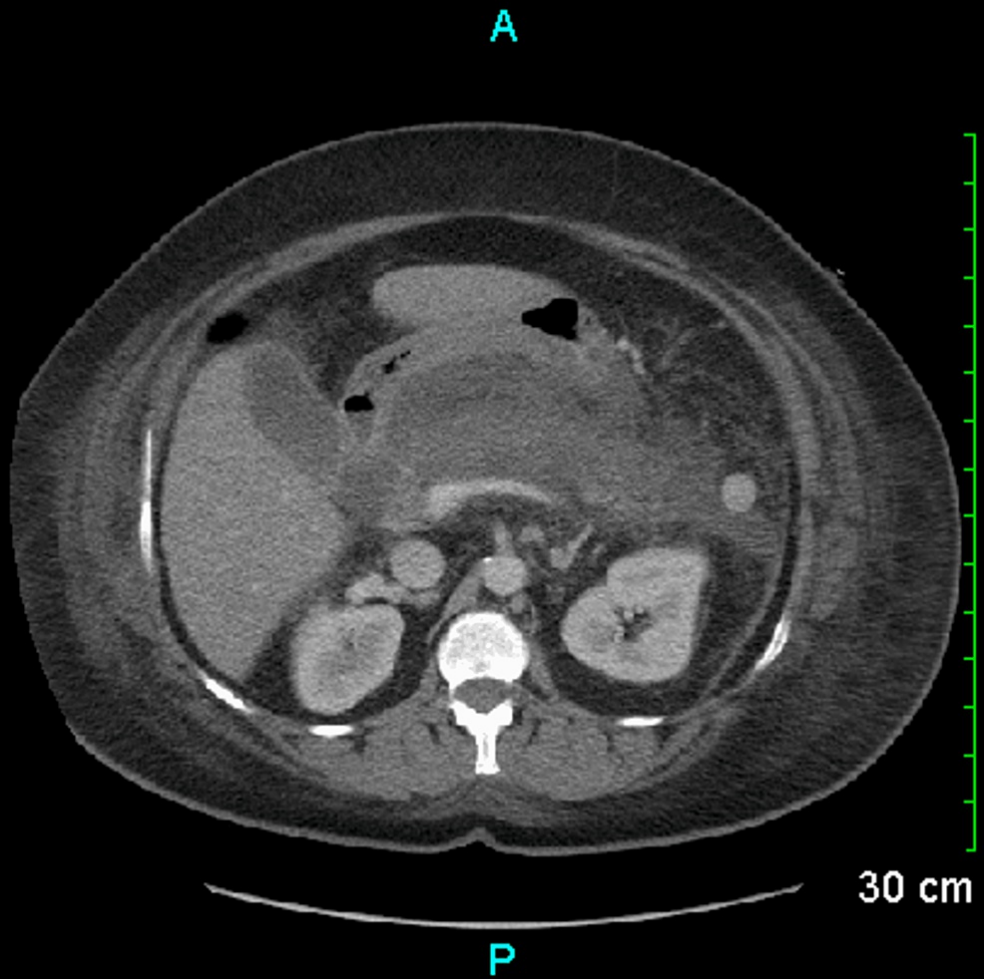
Follow-up CT abdomen on day six of admission. Pancreas with minimal enhancement, concerning necrosis.
